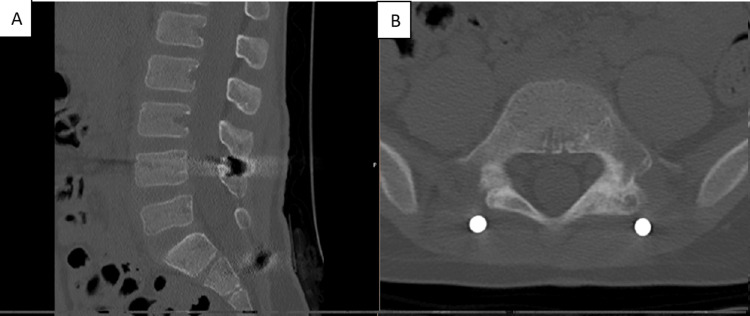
CT scan images at six months postoperatively (A) Sagittal view. (B) Axial view-iliac screws
